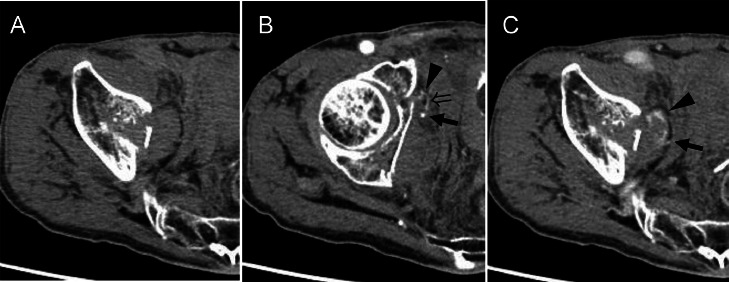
Dynamic contrast-enhanced CT. (A) Noncontrast phase; (B) arterial phase; and (C) equilibrium phase. Compared to the noncontrast phase (A), contrast extravasation (arrowheads) is shown in both the arterial (B) and equilibrium (C) phases. A branch (open arrow) of the right obturator artery (arrow) is situated adjacent to the site of extravasation, suggesting injury or disruption to this arterial branch as the likely source of hemorrhage.
